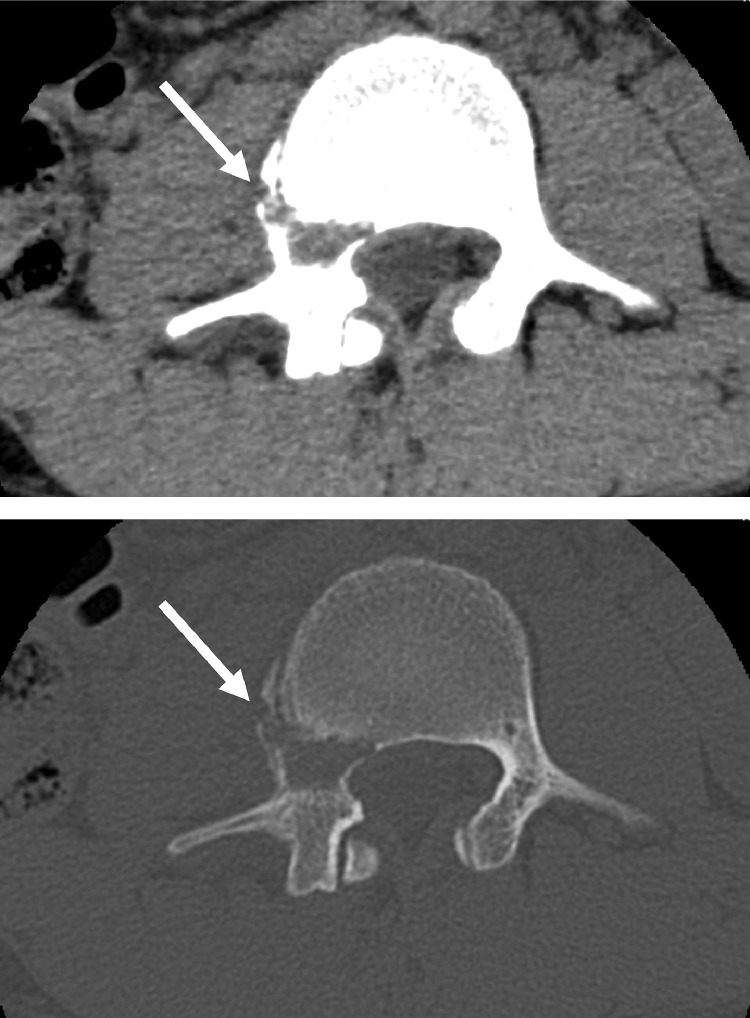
Computed tomography scan showing osteolytic changes in the right pedicle of the third lumbar vertebra.
